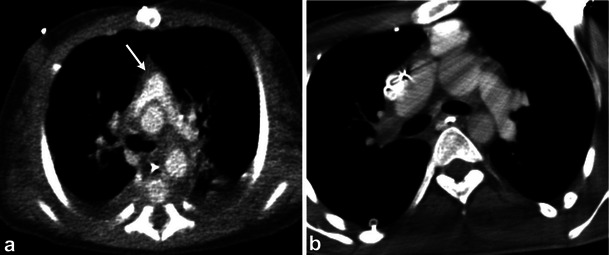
Contrast enhanced axial CT images in a patient with transposition of great vessels demonstrates the characteristic appearance post-arterial switch. The pulmonary arteries (*arrow*) are positioned anterior to the aorta with the left and right main branches draping around the aorta. There is a higher incidence of pulmonary artery stenosis in these patients
